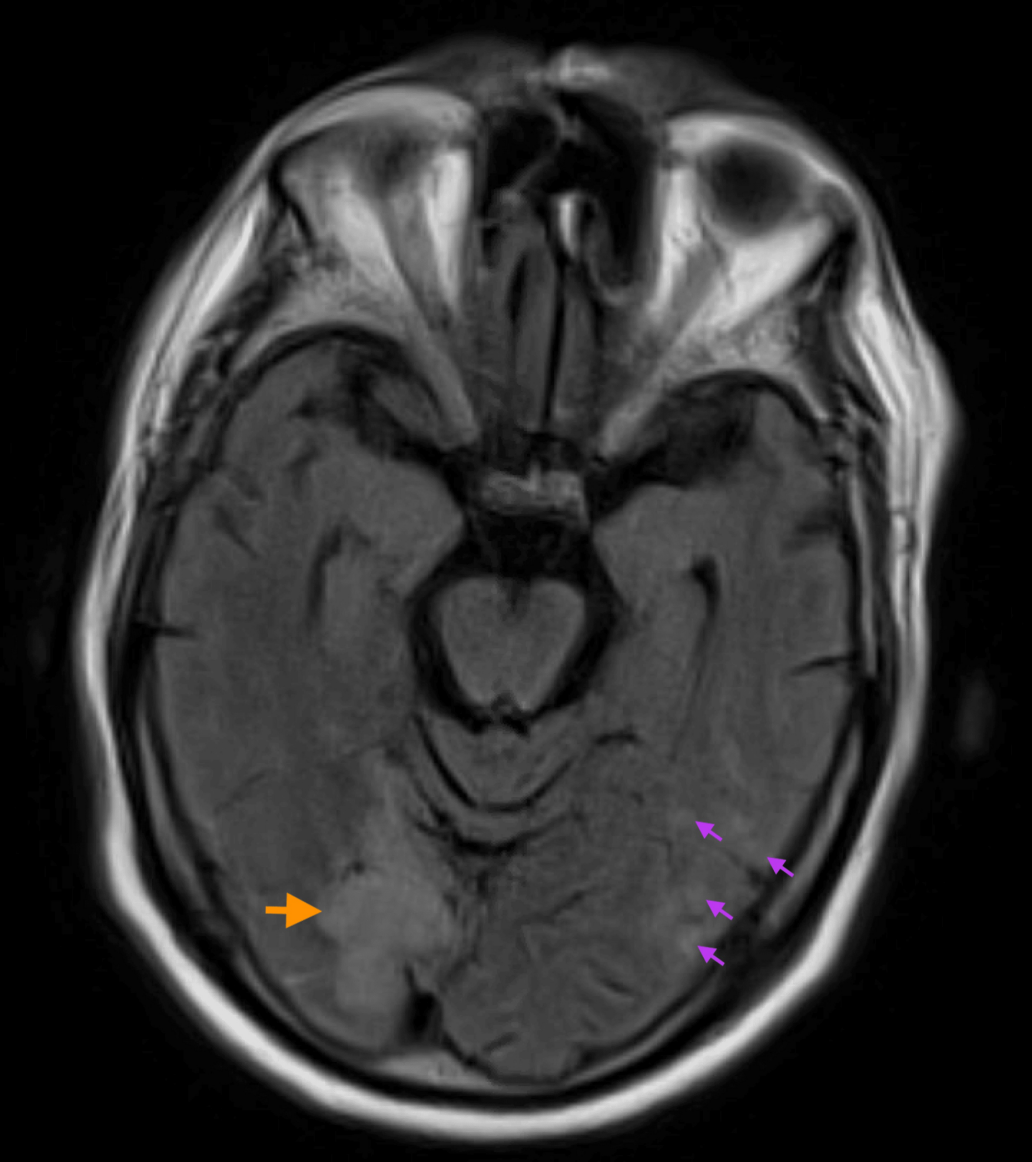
Brain MRI imaging Axial view of the brain MRI showing an area of hyperintensity in in the right occipital lobe (orange arrow) consistent with the finding on the Head CT, in addition to numerous small areas of hyperintensity (purple arrows) signifying acute and subacute infarcts.
